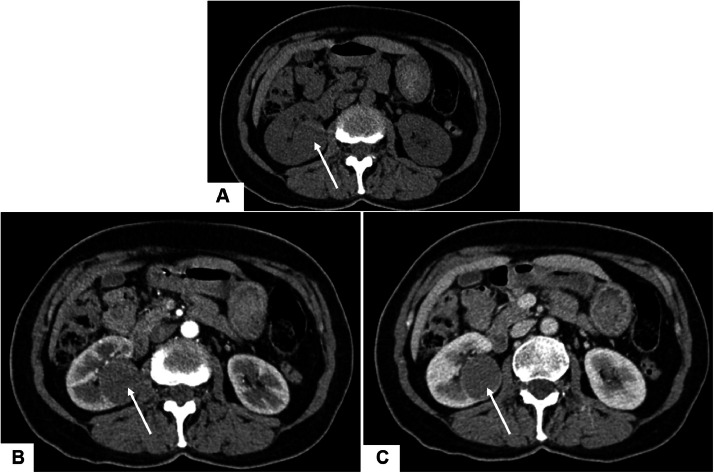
Axial contrast-enhanced CT images of the abdomen: (A) non-contrast, (B) arterial phase, and (C) portal venous phase. A well-circumscribed ovoid mass (arrow) is identified at the lower pole of the right kidney, demonstrating a mean attenuation of approximately 33HU on non-contrast images, 48 HU in the arterial phase, and 53 HU in the portal venous phase, consistent with mild and homogeneous enhancement.
